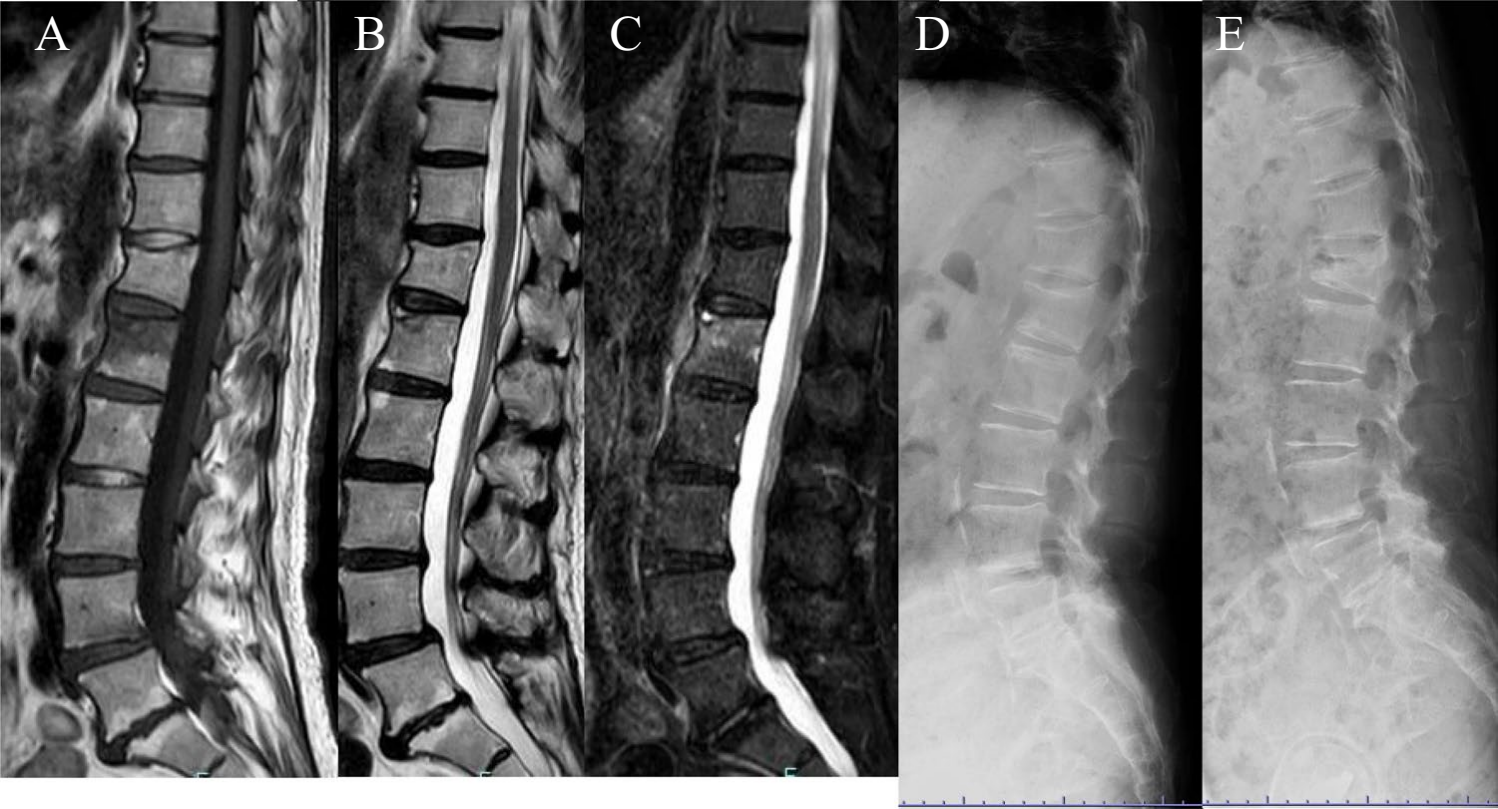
Images of patients with pseudoarthrosis of L1 fracture. MR T1-weighted image (A), T2-weighted image (B), and STIR image (C) at injury. D shows lateral radiogram at injury. E shows lateral radiogram 1 year after the injury
